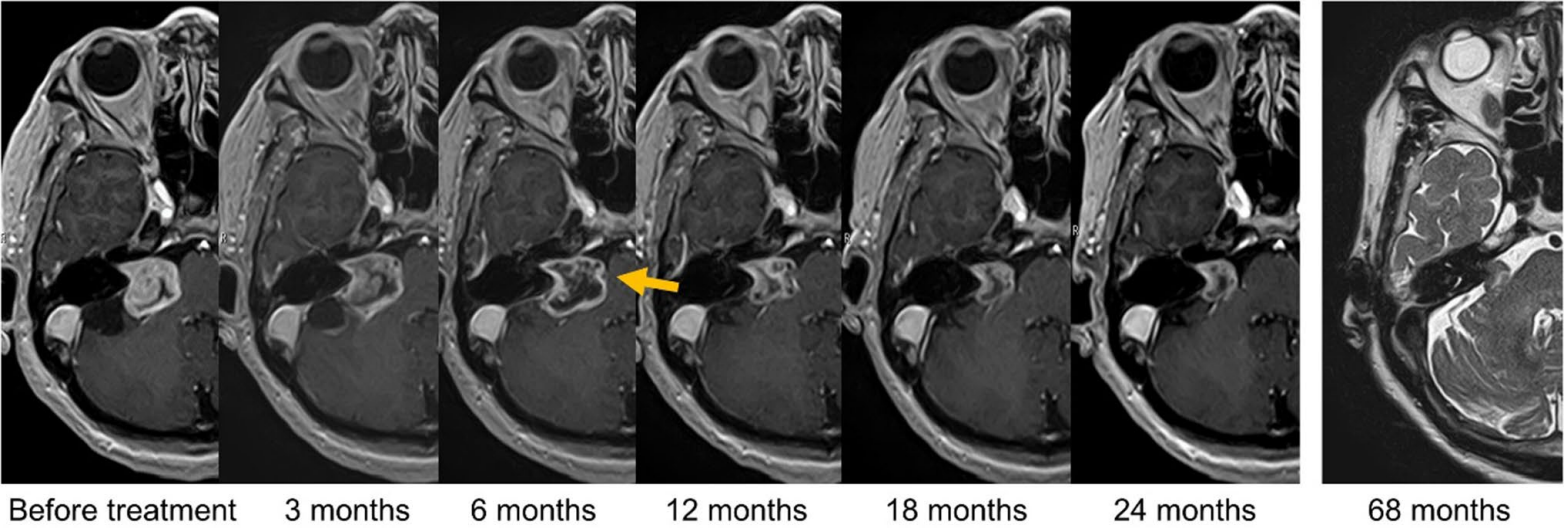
Typical example of a Type A tumor (transient expansion) in a 70-year-old woman. Gadolinium-enhanced T1-weighted imaging shows a notch appearance 6 months after stereotactic radiotherapy. The tumor relaxes and shrinks thereafter. No re-expansion is found within 68 months. A three-dimensional T2-weighted image shows no re-expansion at 68 months after radiotherapy (the last follow-up); no contrast medium was used as the patient did not provide consent
